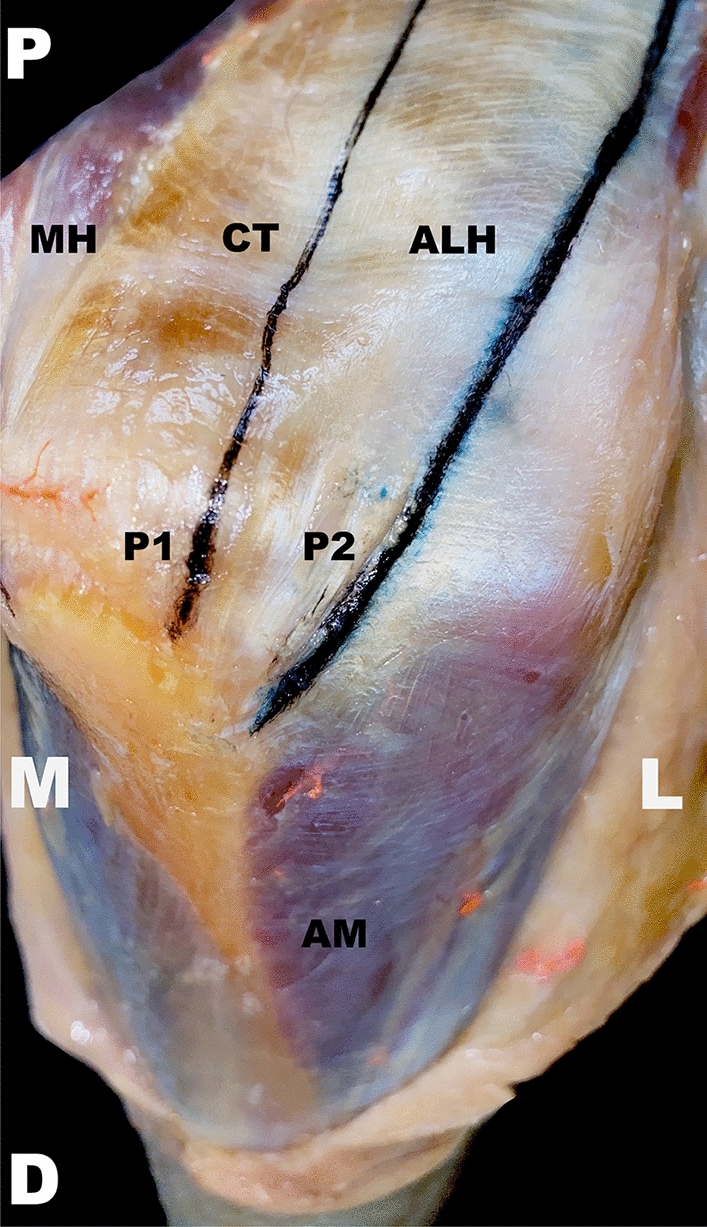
Approaches in a detailed aspect. The approaches are marked in black. The anconeus muscle can be viewed in its entirety. A right upper extremity is pictured in a dorsolateral view. *P1* medially/centrally orientated lateral para-olecranon approach 1, *P2* laterally orientated lateral para-olecranon approach 2, *AM* anconeus muscle, *ALH* aponeurosis of the lateral head, *CT* central tendon, *D* distal, *M* medial, *MH* medial head of the triceps muscle, *L* lateral, *P* proximal
